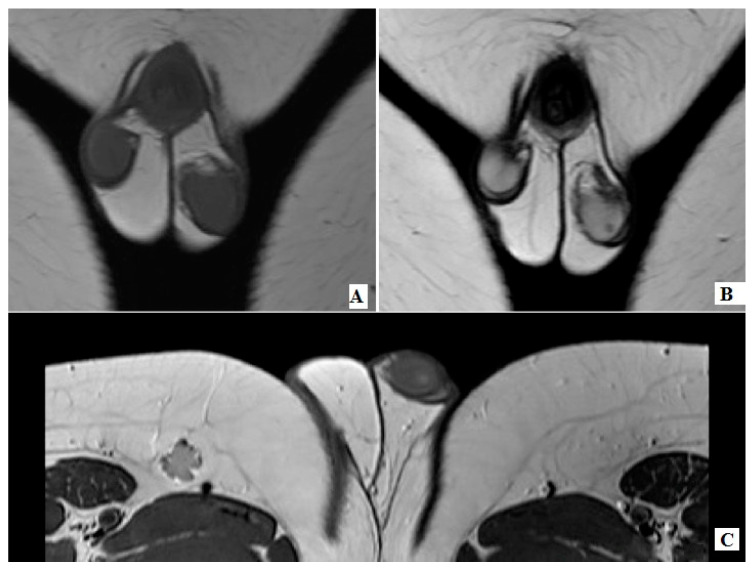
(A) Magnetic resonance imaging (MRI) depicting the lesion as isointense on T1-WI, (B) hypointense on T2-WI, (C) and with intense enhancement after administration of endovenous contrast material.
